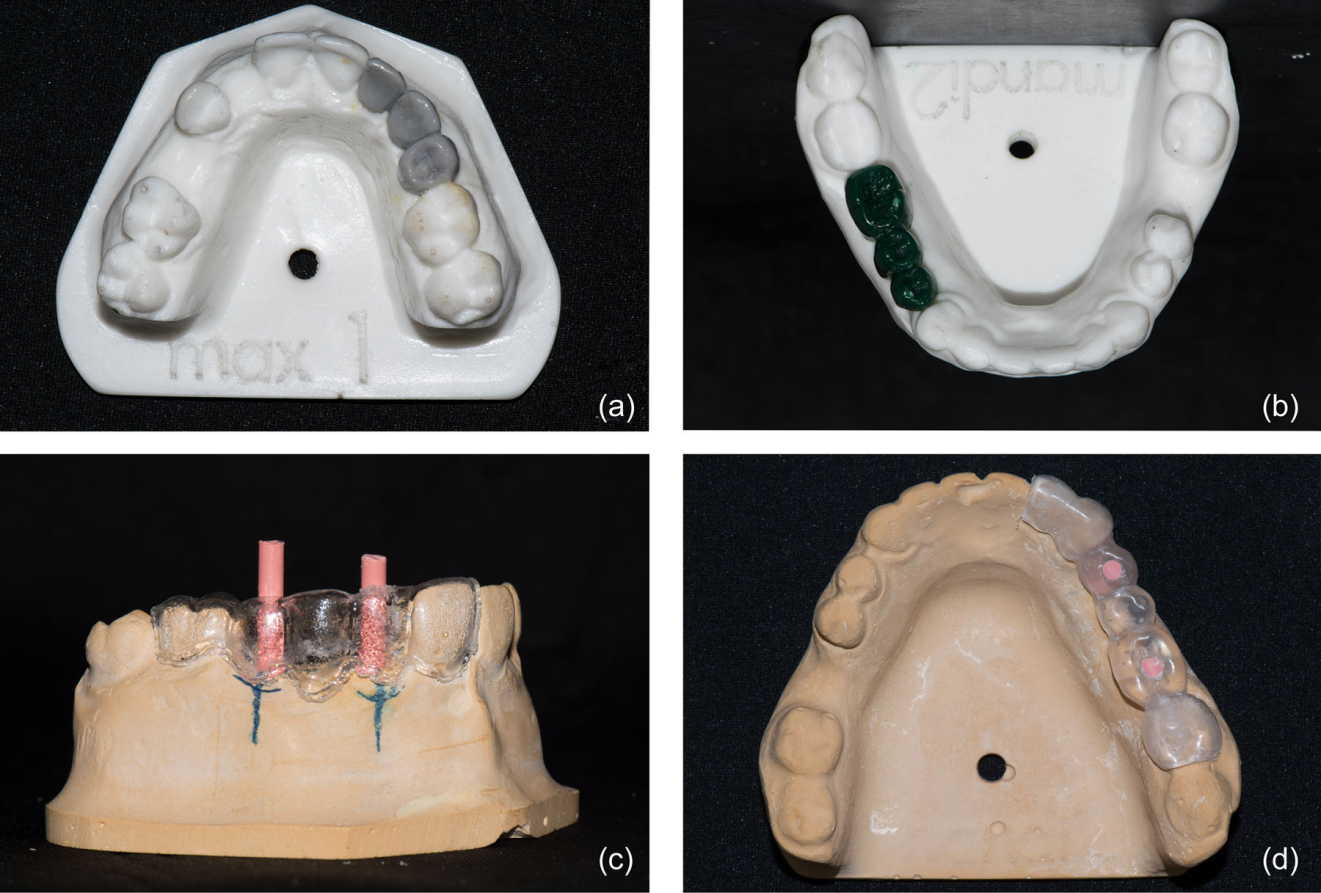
(a) maxillary PMMA model with wax up; (b) mandibular PMMA model with wax up; (c) maxillary model during radiographic guide/stent production—gutta‐percha insertion for planning implant position/axis (d) final radiographic guide/stent on mandibular model.
